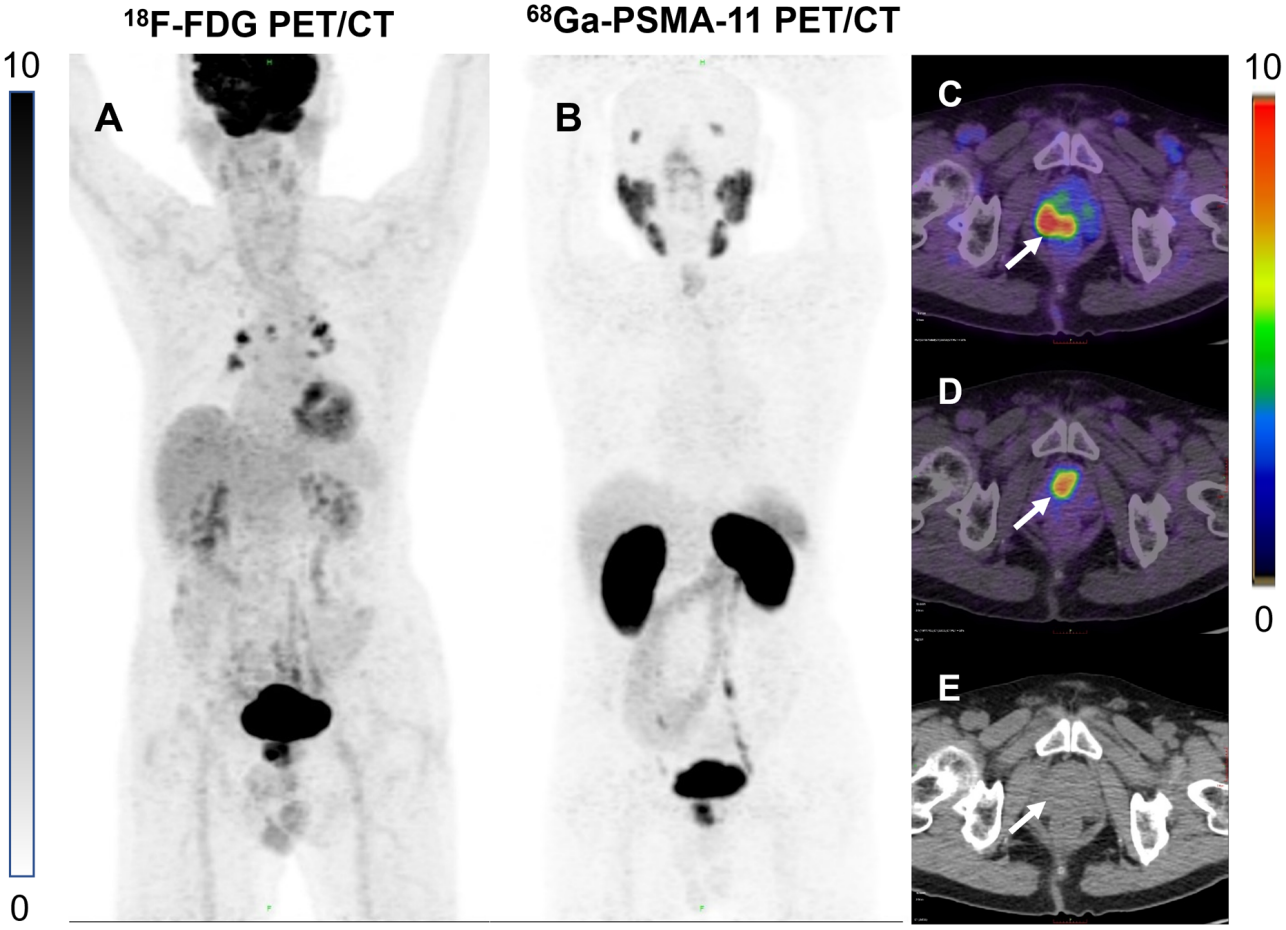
(A) 86-year-old man with a high uptake lesion in the right posterior aspect of the prostate was detected at FDG 3 h delayed imaging [(C), white arrows]. High uptake lesion in the central anterior aspect of the prostate was detected on PSMA 3 h delayed imaging [(D), white arrows]. No obvious lesions were seen on the CT images either (E). (A, B) are MIP maps of whole-body PET scans of FDG and PSMA, respectively.
